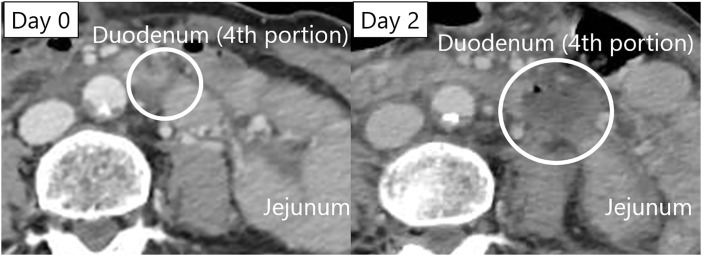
Contrast-enhanced CT findings. Two days after the injury, a CT scan revealed poor contrast (circle) in the intestinal wall of the 4th portion of the duodenum and a very small amount of free air.
